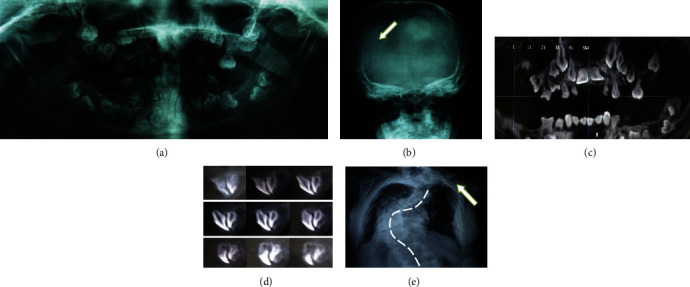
Radiographic evaluation. (a) Panoramic view of the jaws showing multiple unerupted supernumerary teeth. (b) Frontal radiograph showing a delayed closure of sagittal suture and the presence of Wormian bones. (c) Cone-beam radiograph of maxillary and mandibular arches showing a dilacerated root and inverted erupting pathway. (d) Sagittal cone-beam computed tomography section showing an incomplete formation of the left unerupted permanent incisor root. (e) Thorax radiograph showing hypoplasia of the left clavicle.
